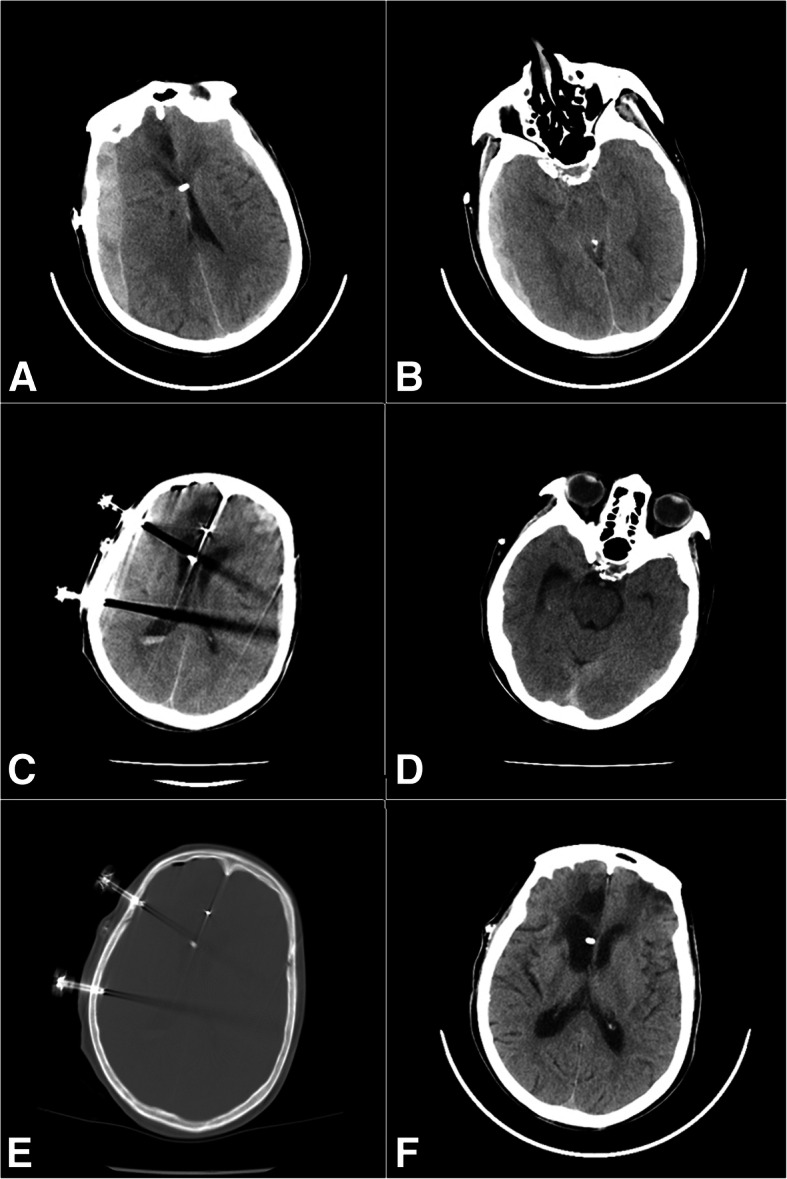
Preoperative brain CT scan showed a large traumatic acute subdural hematomas (TASDH) in the right frontal-temporal-parietal region (a, b). Brain CT scan revealed there was a small amount of hematoma in the right subdural space and expansion (c, d). 1 day after surgery. CT showed the puncture needle passing through the skull and dura and just reaching the edge of the hematoma cavity (e). A follow-up brain CT scan 6 months later after TDC (f)
